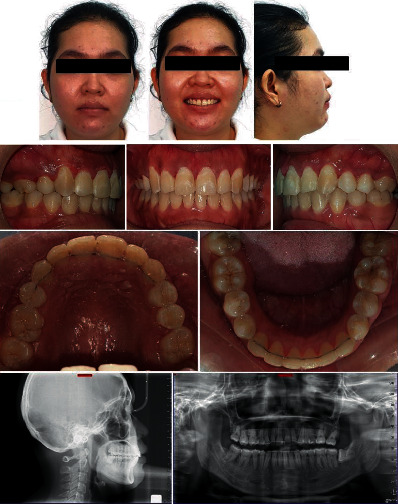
Posttreatment extraoral and intraoral pictures with radiographs after gingivectomy.
